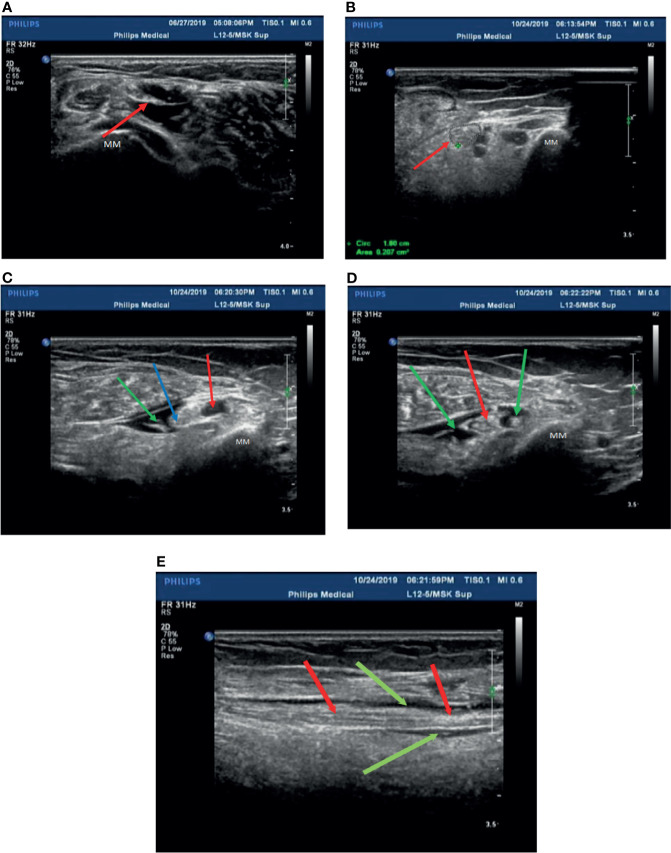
Patient 2 was treated with mecobalamin by ultrasound-guided hydrodissection injection at the malleolus medialis of the posterior tibial nerves (A–E). (A) A normal posterior tibial nerve is shown as a clear neural boundary (cribriform network), normal internal echo (the posterior tibial nerve is below the red arrowhead). (B) The posterior tibial nerve of patient 2 was thickened with a fuzzy boundary in the medial malleolus (the red arrowhead indicates the posterior tibial nerve). (C) Injection with 3 ml saline at the medial malleolus of the posterior tibial nerve (the posterior tibial nerve at the red arrowhead, the point of a needle at the blue arrowhead, and the green arrowhead points to saline after injection). (D) The posterior tibial nerve was injected with mecobalamin at the medial malleolus after injection in the transverse section, and it can be seen that the solution is evenly wrapped around the posterior tibial nerve (red arrowhead pointed to the posterior tibial nerve, saline + mecobalamin at the green arrowhead). (E) The medial malleolus of the posterior tibial nerve after injection with mecobalamin in the longitudinal section, it can be seen that the fluid evenly covers the posterior tibial nerve (the red arrowhead points to the posterior tibial nerve, and saline + mecobalamin at the green arrowhead). MM; Medial malleolus.
